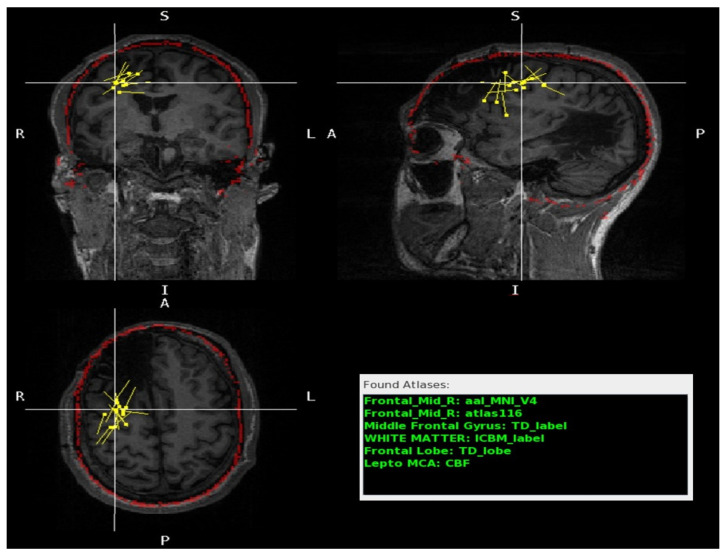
A patient with right frontal and temporal cystic encephalomalacia. The MRI scan is shown in three different planes: coronal (top left), sagittal (top right), and axial (bottom left). The red outline indicates the contour of the skull model overlay and demonstrates satisfactory patient anatomy registration. The yellow dots represent the dipoles modeled. The crosshair dipole is close to the cavity but in a preserved anatomic area. The Found Atlases section (bottom right) lists the different brain atlases and the corresponding labels (green) for the crosshair dipole. The atlas labels matched the radiologists’ labels with minimal discordance, predominantly in areas at the border of the cavity. The atlas labels matched the radiologists’ label, with minimal discordance, predominantly in areas at the border of the cavity. In Found Atlases, the abbreviation is as follows: Frontal Mid, middle frontal gyrus.
